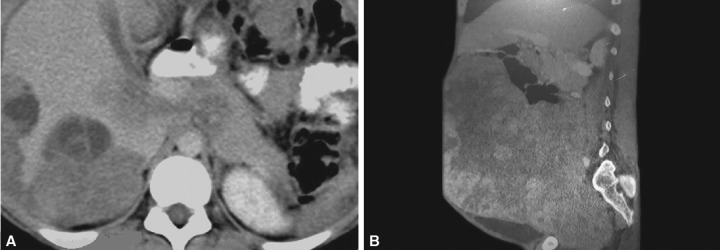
(A) Computed tomography (CT) scan (transverse view) showing scalloping of visceral surface due to compression by the peritoneal implants and (B) CT scan (lateral view) showing hyperdense mucinous ascites filling the peritoneal, appendix not visualized
